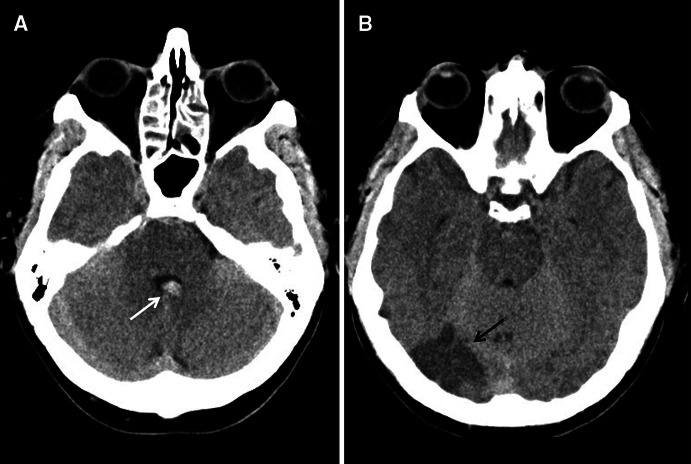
Axial CT scans showing acute intraventricular hemorrhage (a, *white arrow*), and right posterior cerebral artery territory infarction (b, *black arrow*)
